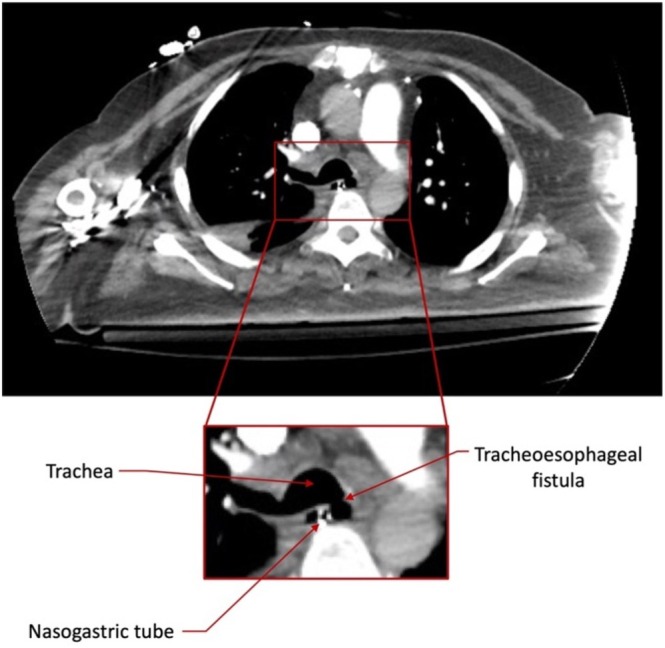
Computed tomography (CT) scan of the chest demonstrating a tracheal defect at the entrance of the left mainstem bronchus.
